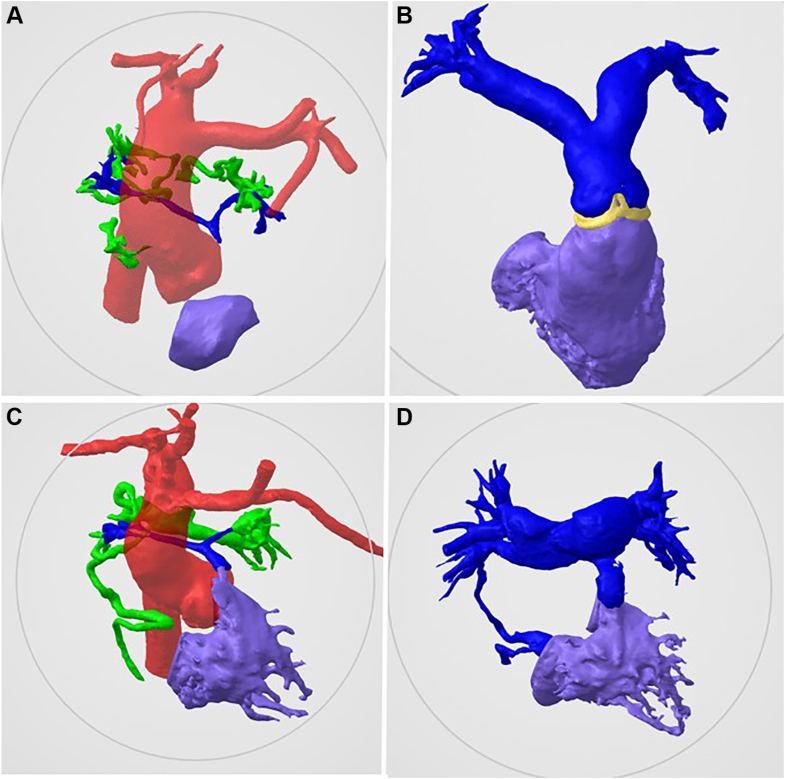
Three-dimensional reconstruction from computed tomography scan demonstrates interval growth of the pulmonary artery (PA) at birth and at the latest follow-up. (A, B) Case 1 with pulmonary atresia with intact ventricular septum, a hypoplastic right ventricle, severely hypoplastic native PA, and tiny major aortopulmonary collateral arteries. (C, D) Case 2, a patient with pulmonary atresia with ventricular septal defect and major aortopulmonary collateral arteries. Color coding: RV (purple), PA (blue), aortopulmonary collaterals (green), and aorta (red).
